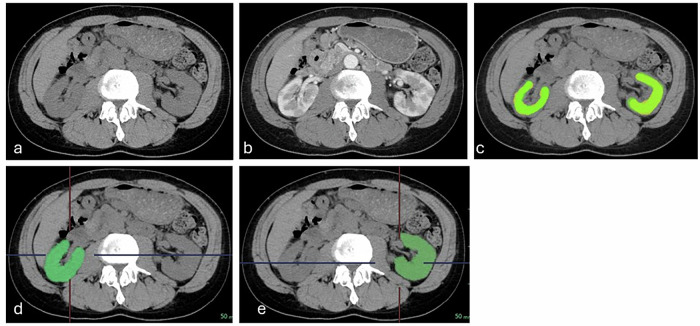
CT of an IgG4-RKD–positive case (male, 55 years). a Unenhanced CT of the kidney; b CE-CT of the kidney showing low-attenuation nodules in both kidneys; c ROIs for texture extraction by MH (yellow) and JI (green); d, e segmentations (green) of the right and left kidneys by MH. CE-CT, Contrast-enhanced computed tomography; CT, Computed tomography; I gG4-RKD, Immunoglobulin G4-related kidney disease; ROI, Region of interest
